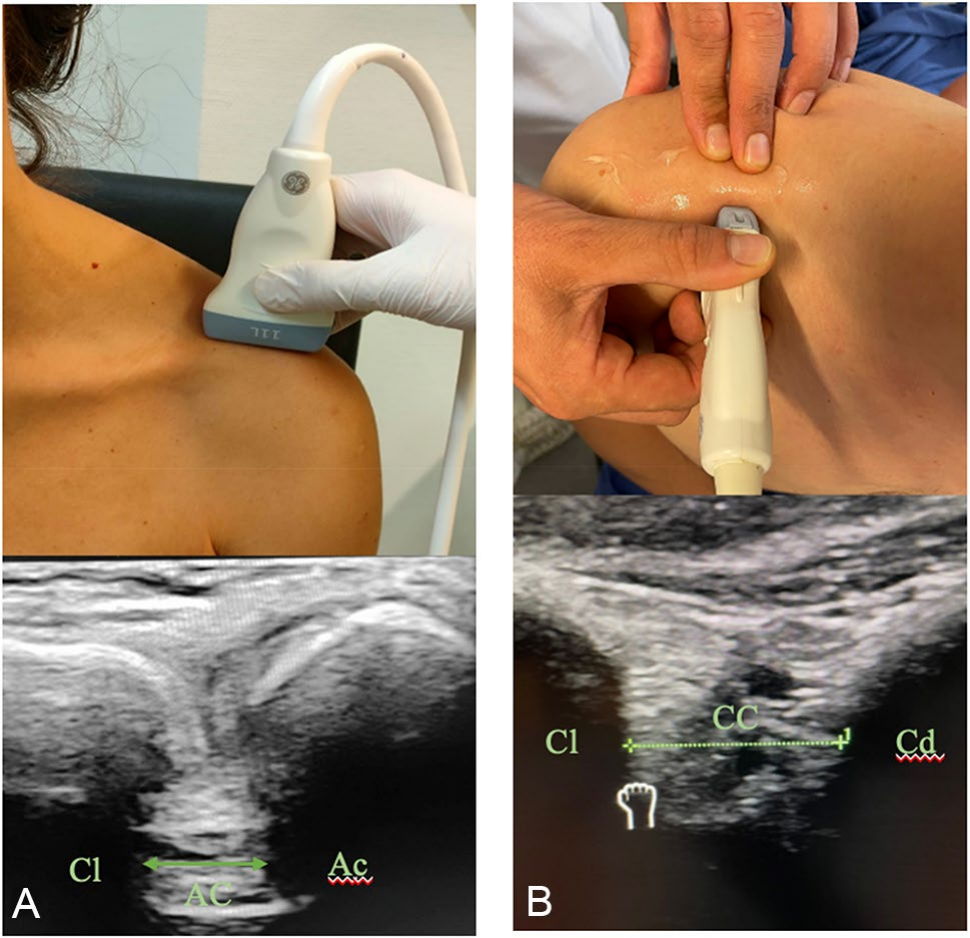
Sonographic assessment of AC (A) and CC (B) distance following ACJ reconstruction. *Cl* clavicle, *AC* acromioclavicular distance, *Ac* acromion, *CC* coracoclavicular distance, *Cd* coracoid
